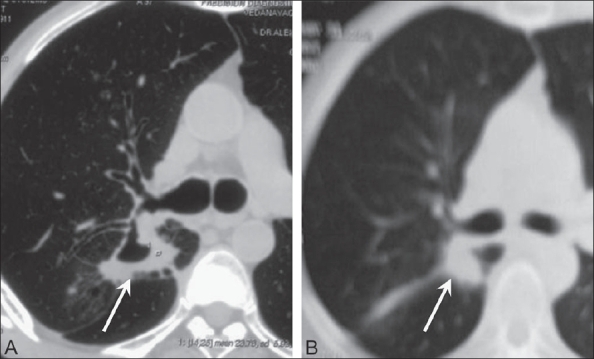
Bronchogenic carcinoma. Axial CT scan images obtained 3 months (A) and 9 months (B) following ablation of the same lung mass shown in Figure 5. The lesion shows significant reduction in size with cavitation (arrow) at 3 months and with further reduction (arrow) at 9 monthsb. Contrast enhancement: No central or peripheral enhancement is expected at any time after ablation [Figure 7].
